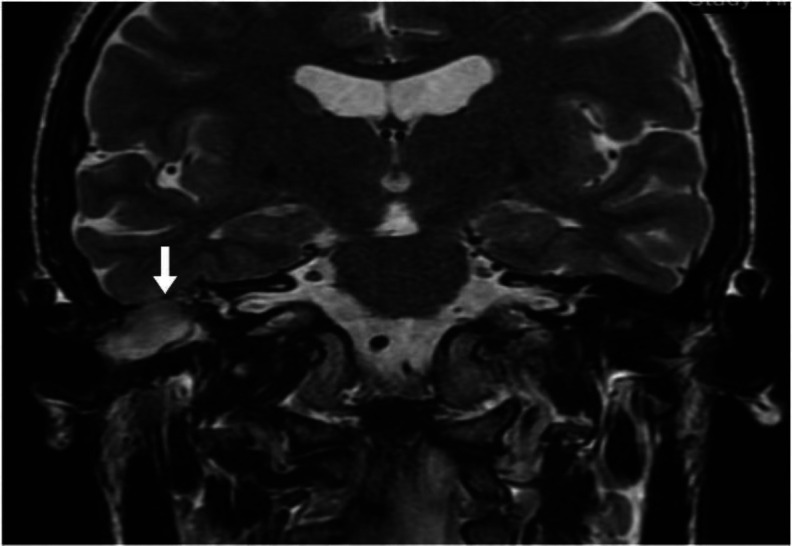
MRI imaging. Coronal view. Brain tissue herniation is identified on the right side
